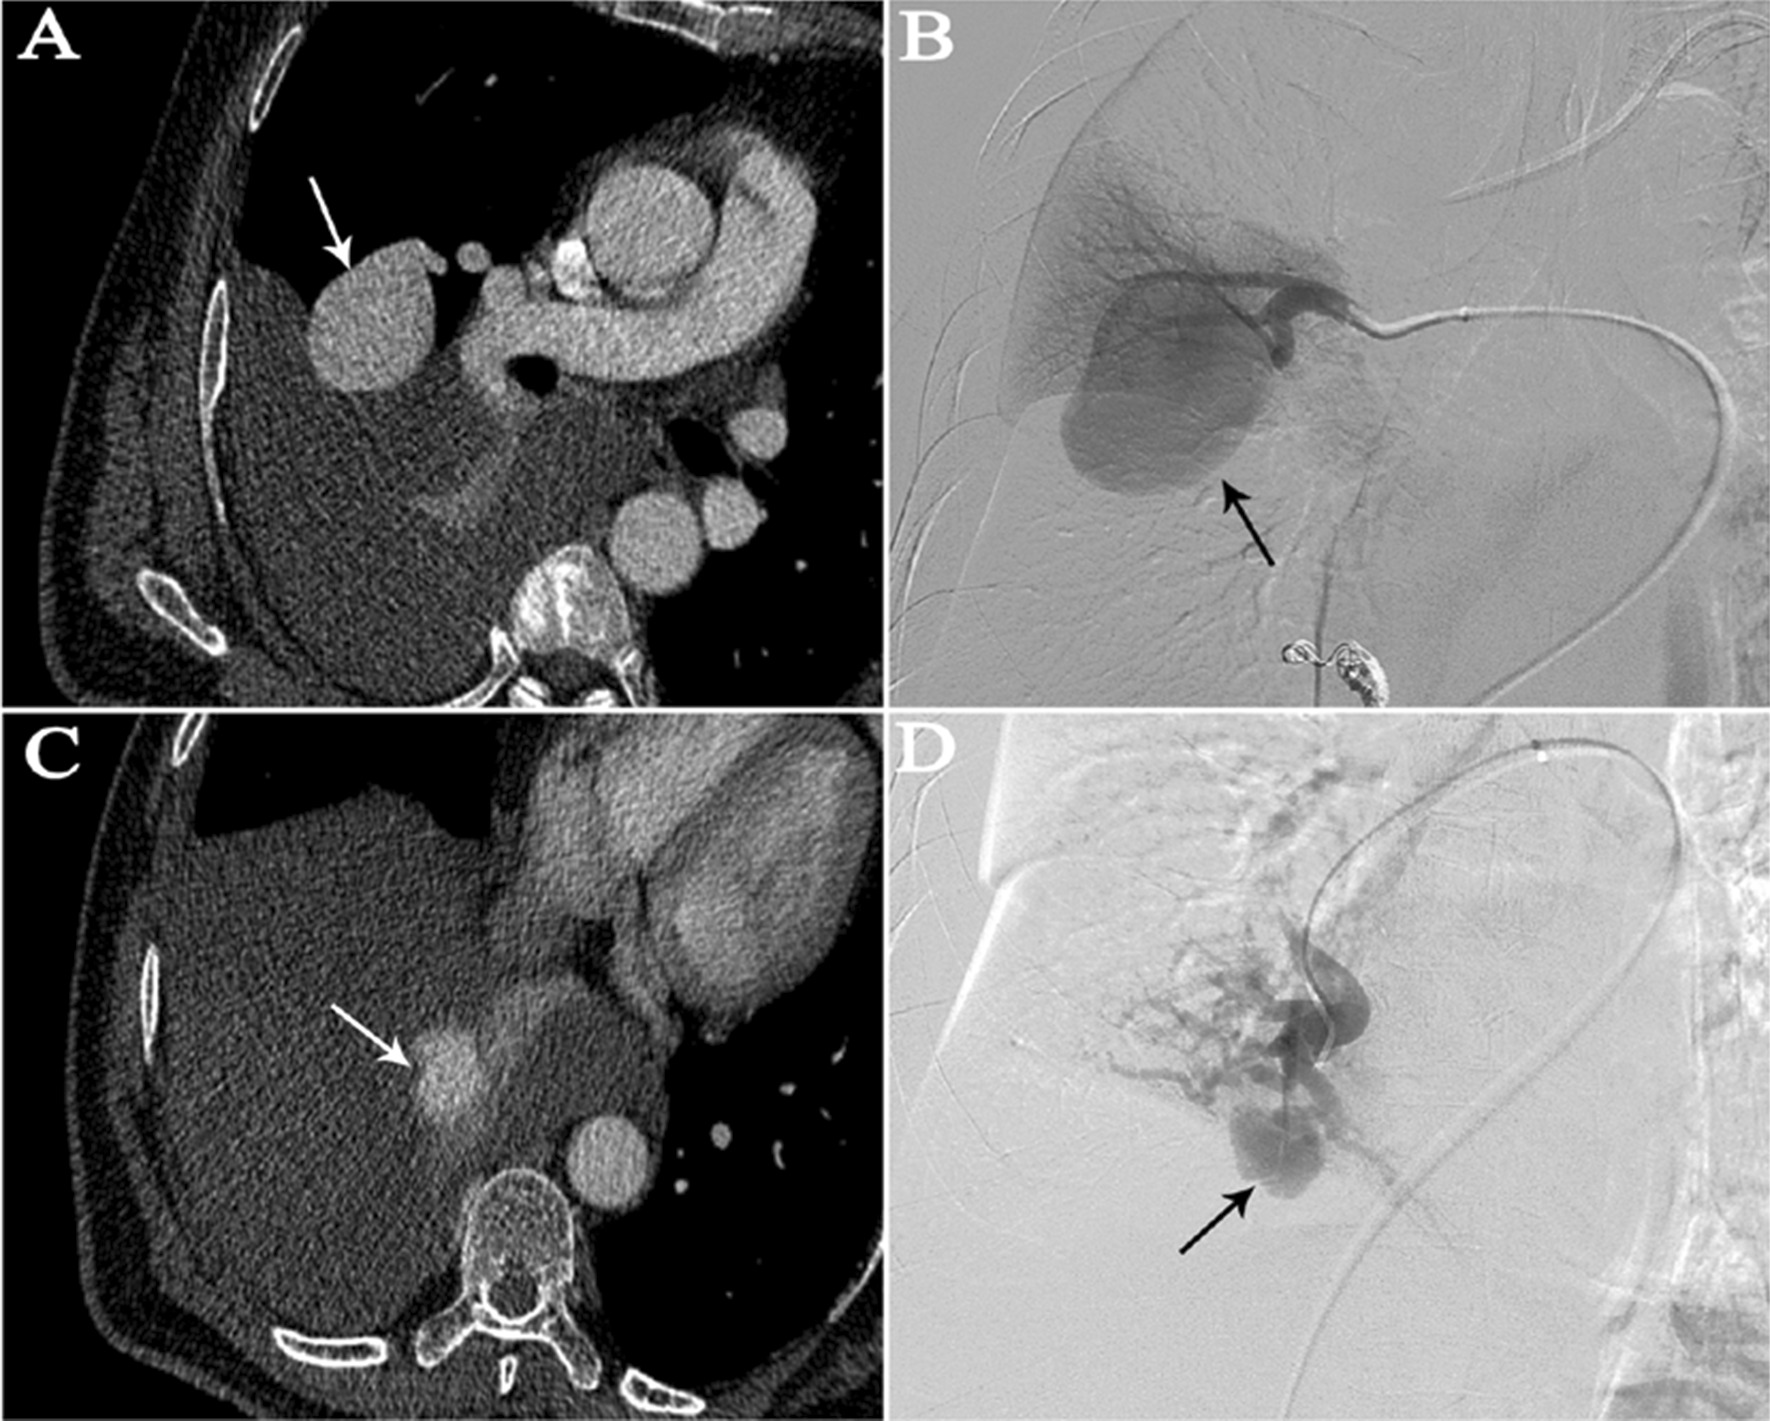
Unilateral multiple PAVMs in a 52-year-old man with haemothorax. A, B one smooth PAVM located in the interlobar pleural area was not considered to be ruptured based on its appearance on multi-detector CTA and pulmonary angiography (arrows). C the ruptured lesion located in the subpleural area manifested as an “anomalous bulge” on CTA (arrow). D this characteristic presented as the “double shadow sign” on angiography, as indicated by the black arrow
